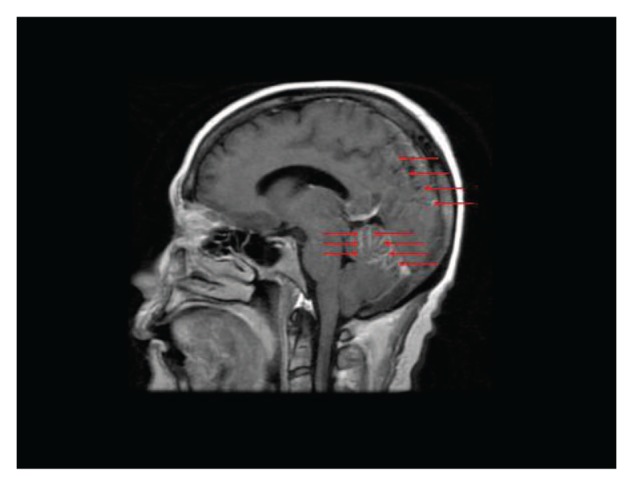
Saggital T1-weighted postcontrast magnetic resonance image of brain demonstrating diffuse leptomeningeal enhancement involving both cerebellar hemispheres, the superior cerebellar vermis, and parieto-occipital lobes, as indicated by the red arrows.
